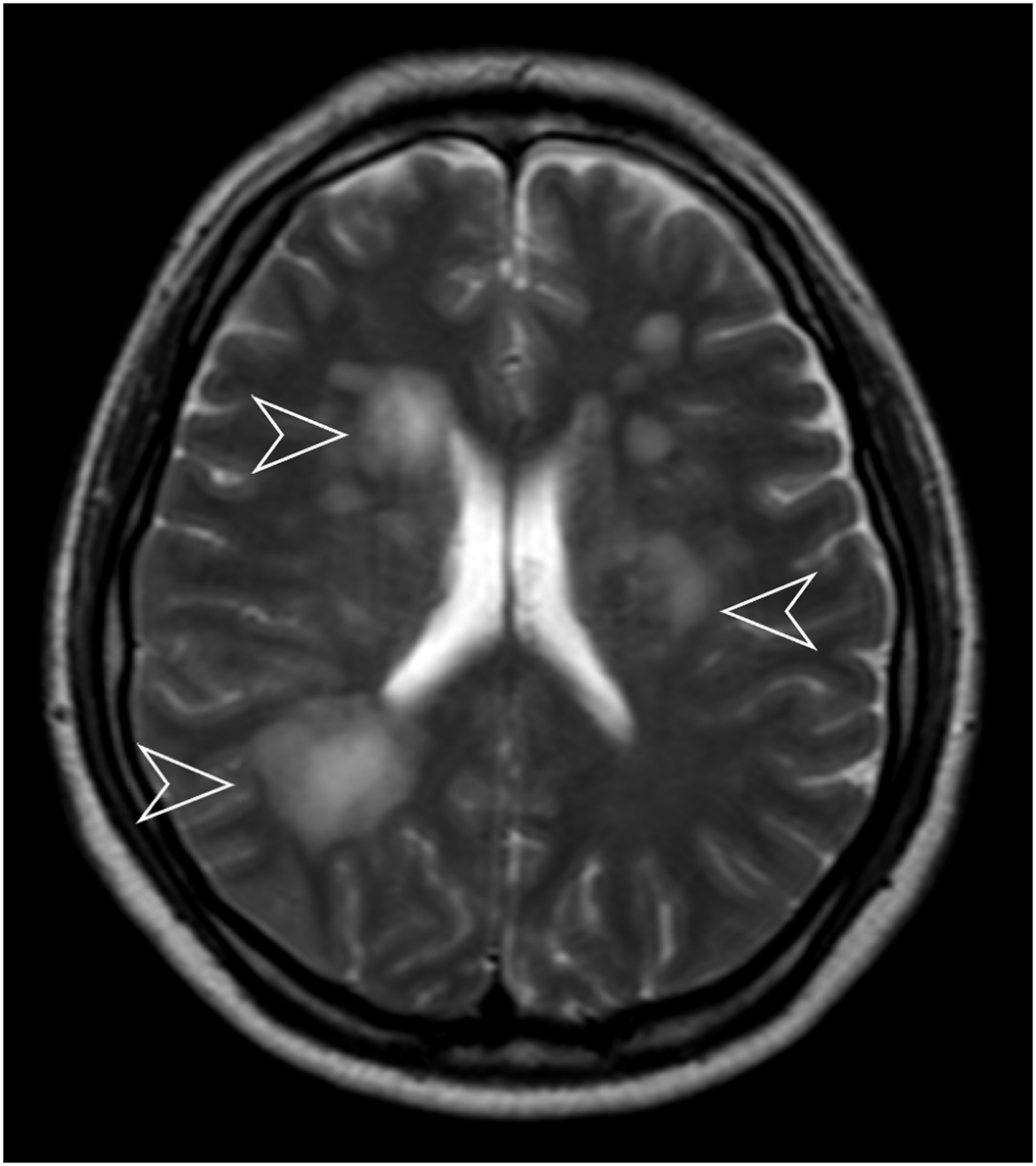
Axial T2 magnetic resonance imaging showing high T2 signal changes that are multifocal and bilateral multifocal bilateral high T2 signal changes in the periventricular deep white matter (arrowheads).
